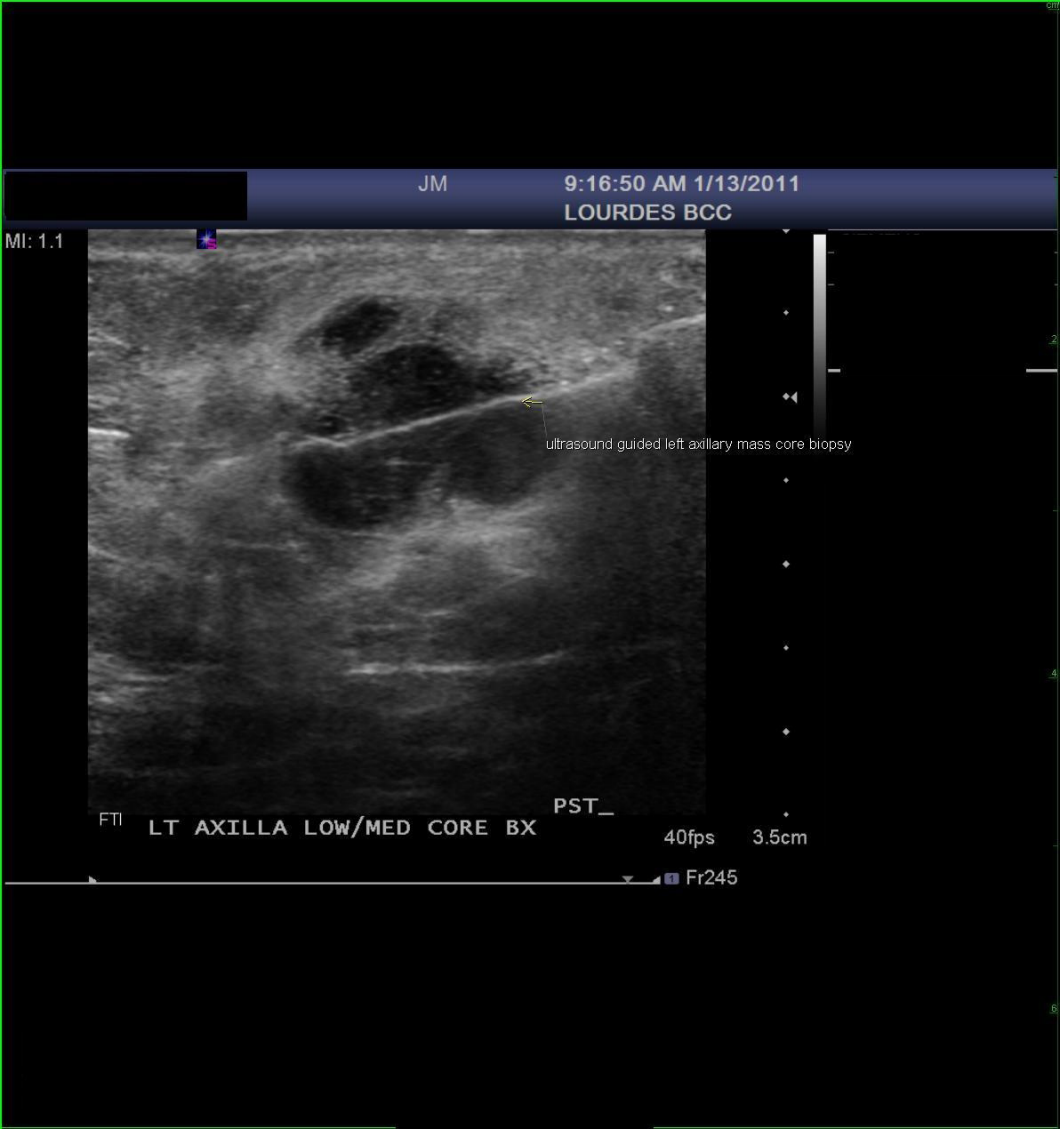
The image shows the ultrasound guided core biopsy of the left axillary mass.
